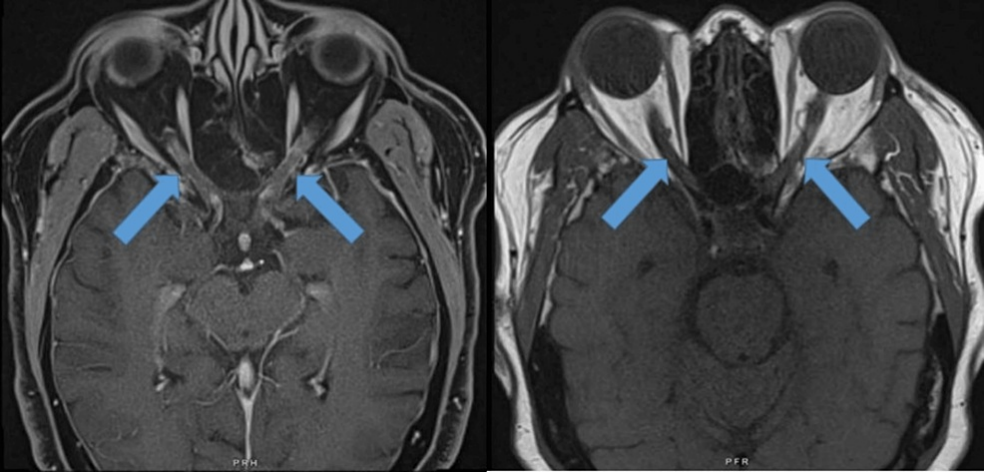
Axial T1 and T2-weighted orbital MRI with fat suppression showing the mildly increased signal intensity of bilateral optic nerves and subtle optic nerve enhancement.
